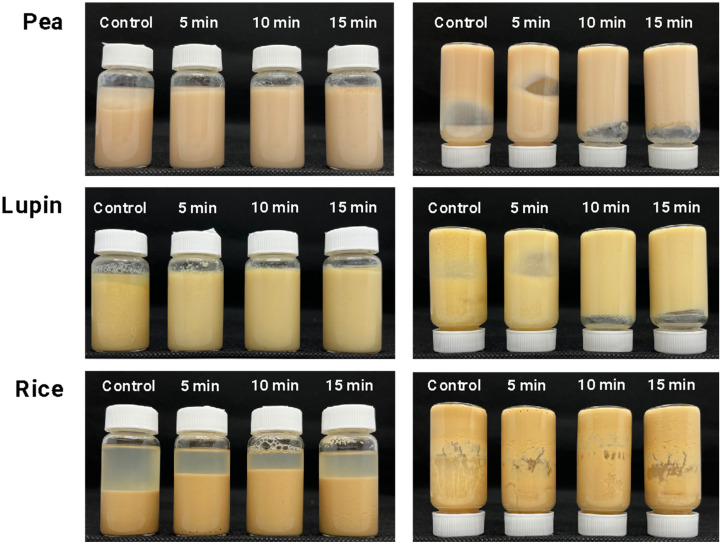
Photographs of gel formation of plant proteins treated with different times of ultrasound.
